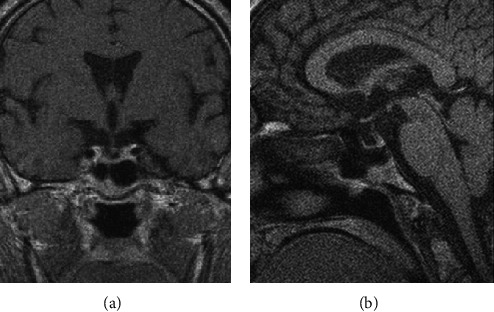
Bulky tongue reaching to the back surface of oropharynx causing obliteration of communication between nasopharynx and oropharynx (MRI of cervical region and cranium: (a) coronal view; (b) sagittal view).
